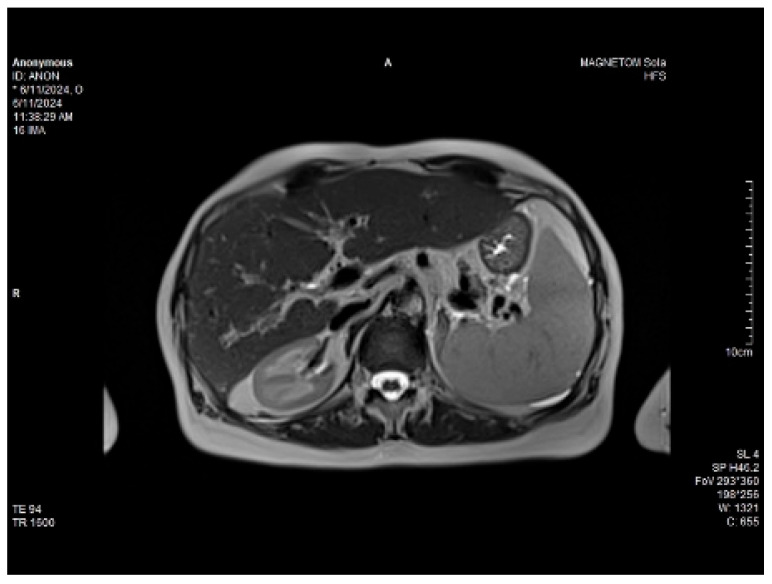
MRI featuring perihepatic and perisplenic ascites.
